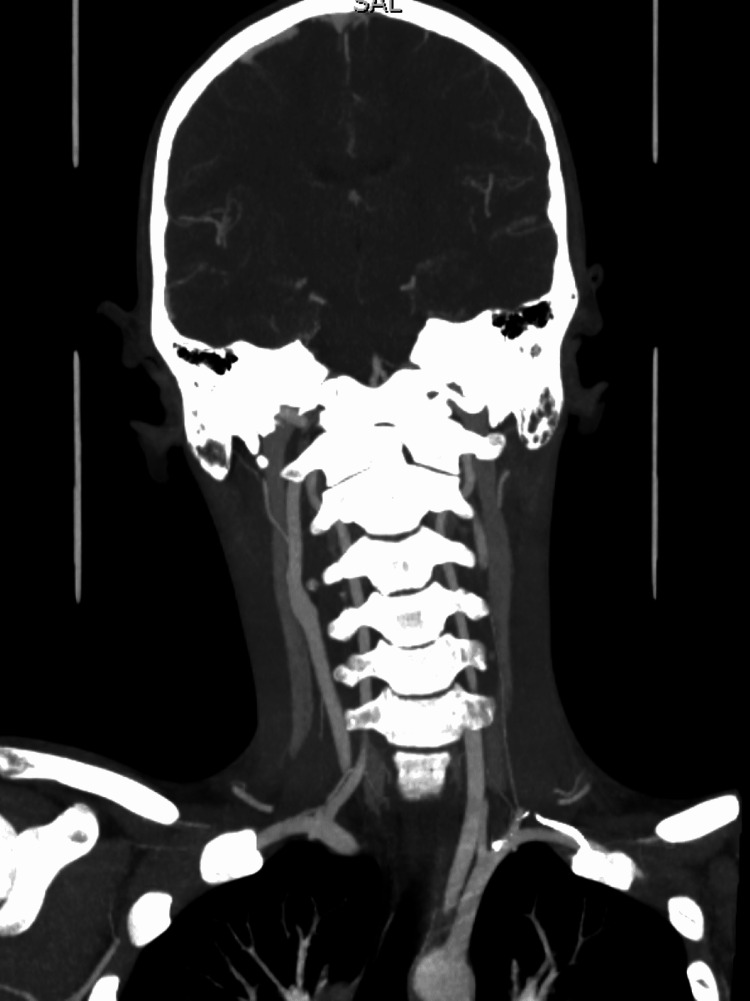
CT angiogram of head and neck. No carotid artery dissection was identified.
